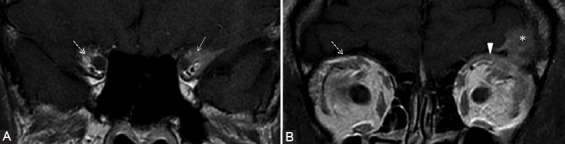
Patient with the left lacrimal gland adenoid cystic carcinoma operated for 6 years, presented for numbness of the forehead. There is tumoral relapse at the level of superior rectus and levator palpebrae muscle. The coronal contrast-enhanced T1-weighted FSE (A, B) demonstrates CE of the fat pad around the CN V1 in the orbital apex (arrow) and fat pad obliteration superior to the levator palpebrae muscle (arrowhead) in the expected location of the V1 branches, compared to their normal appearance on the right side (dashed arrows). The findings are suggestive of PNS along V1. The left frontal cerebral metastasis (*) is also noticed.
